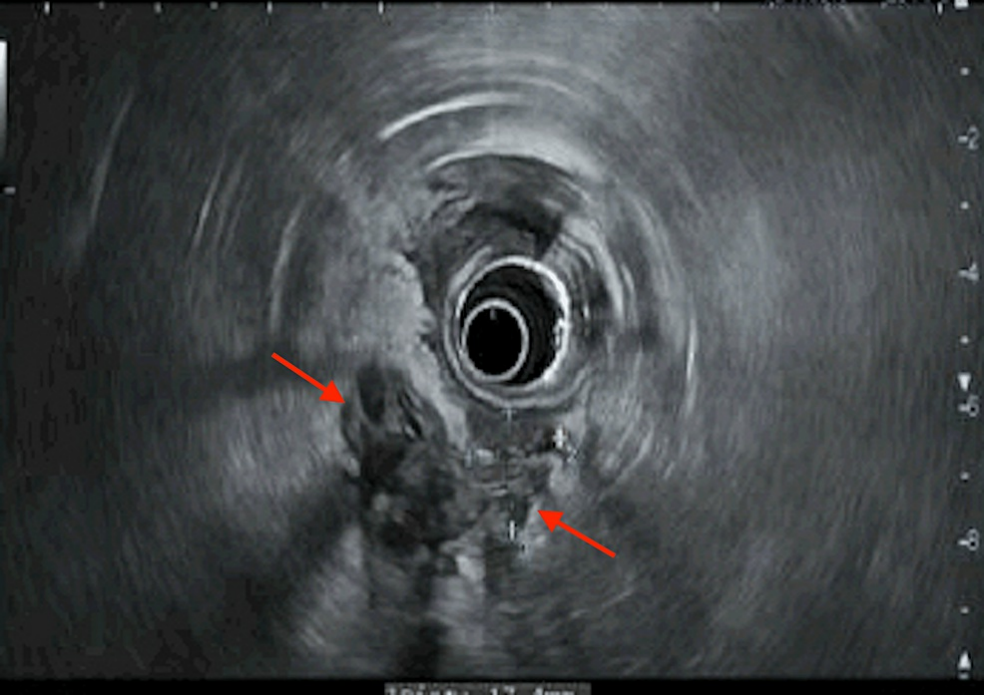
Patient's third EUS demonstrating ampullary mass, which was biopsied and showed adenocarcinoma. EUS: Endoscopic ultrasound
